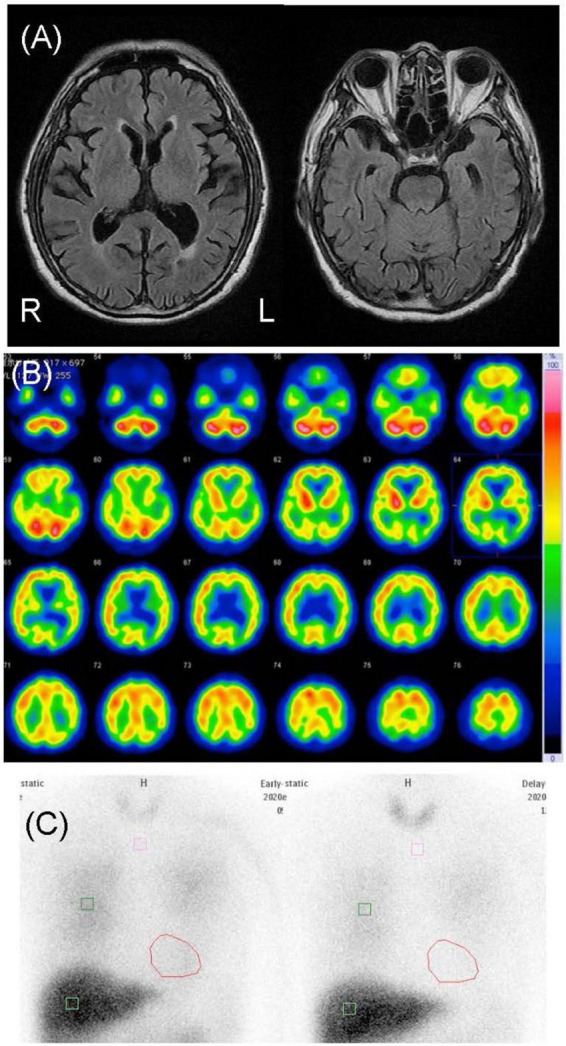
Neuroimages of case 1. (A) Cranial magnetic resonance images revealed diffuse mild atrophy including the bilateral hippocampus. (B) N-isopropyl-p-[^123^I] iodoamphetamine single-photon emission computed tomography revealed hypoperfusion in the bilateral occipital and parietal lobes. (C) Cardiac [^123^I]-metaiodobenzylguanidine scintigraphy revealed heart-to-mediastinum ratios of 1.55 and 1.26 in the early and delayed phases, respectively.
